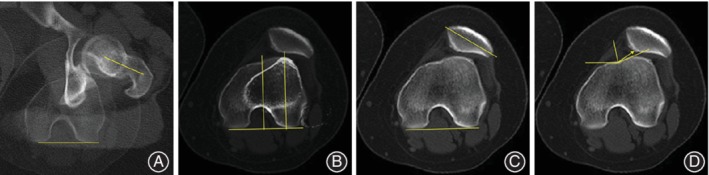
The preoperative measurements under axial CT images. (A) Superimposed images in the sections of the femoral neck and bilateral femoral condyle showing the femoral anteversion angle. (B) Superimposed images in the sections of the bilateral femoral condyle and tibial tuberosity showing the tibia tuberosity‐trochlear groove distance. (C) patellar tilt. (D) congruence angle.
